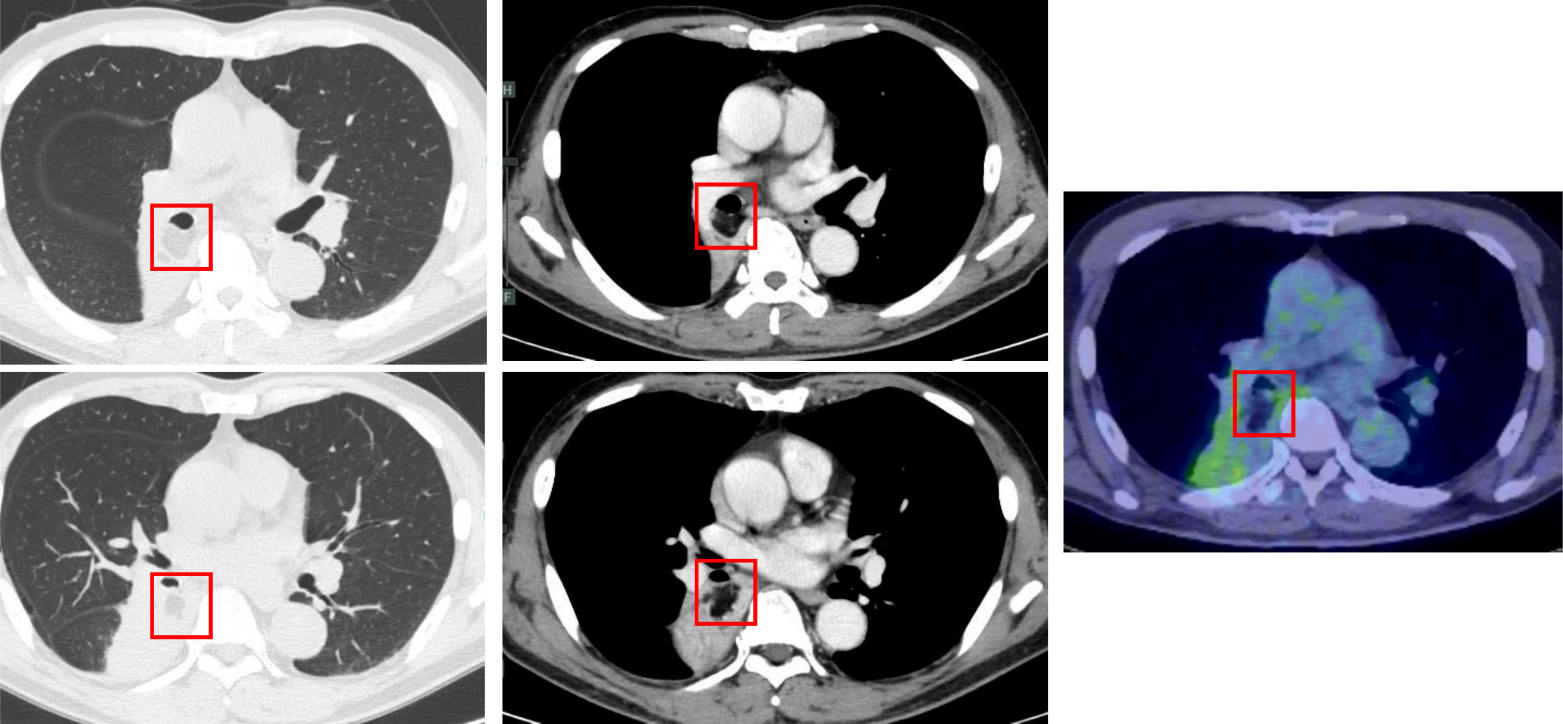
Chest computed tomography and positron emission tomography‐computed tomography at first visit.
